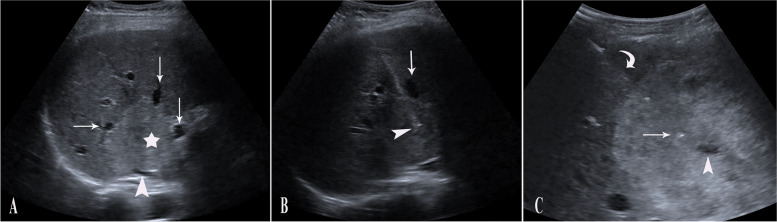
A Transverse liver ultrasound scan demonstrating a hepatic venous malformation (HVM) (marked by a star) that is compressing the inferior vena cava (arrowhead) and hepatic veins (arrows). B A 22-gauge Chiba needle (arrowhead) is passing between hepatic veins (red arrow) into the haemangioma, with a significant portion of normal liver tissue transgressed to reduce the risk of bleeding. C Another patient with HVM during ultrasound-guided access, in which the intervening normal liver parenchyma is notable (curved arrow), and the needle tip (red arrow) is slightly away from the cystic area (arrowhead)
